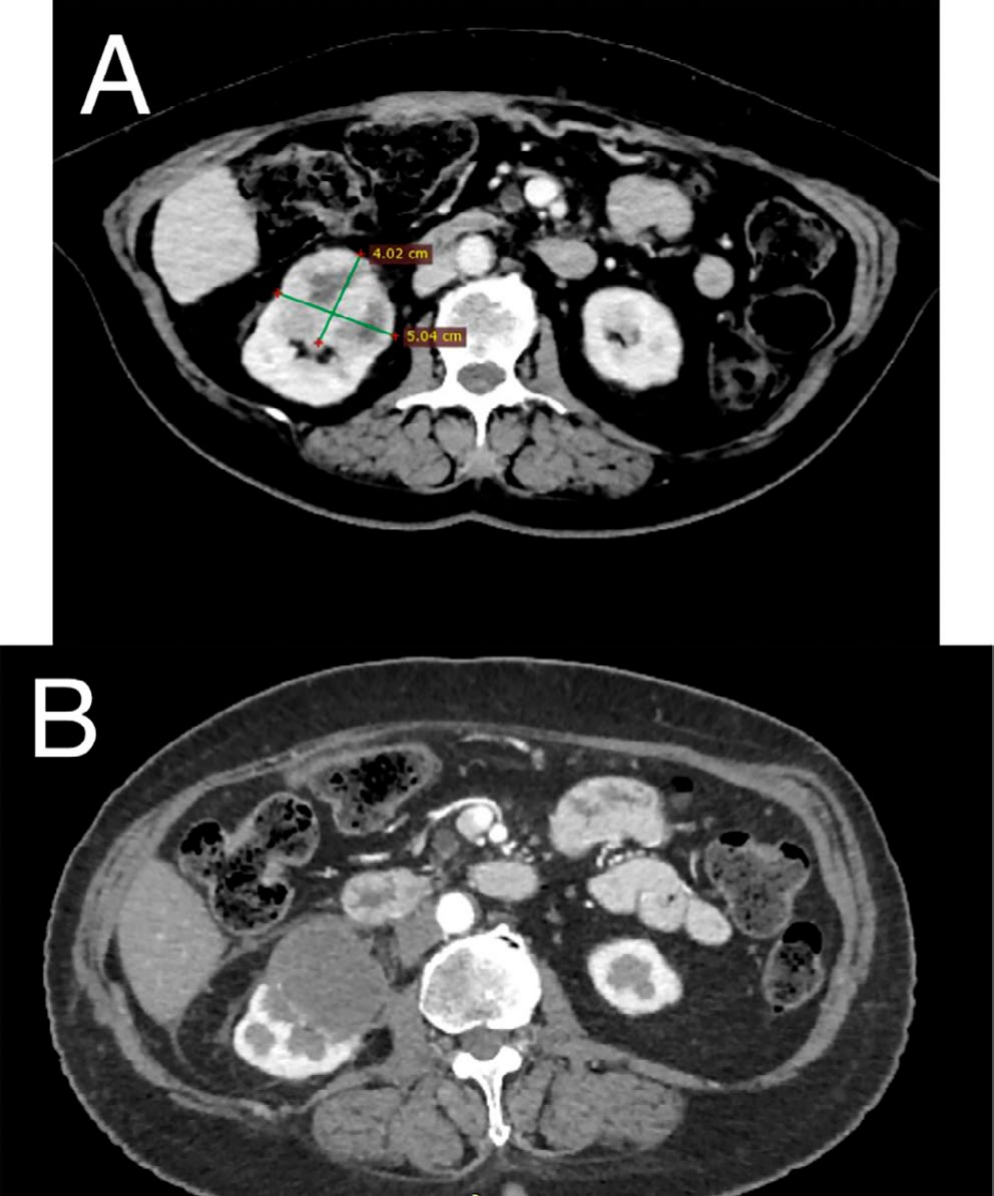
CT images before (A) and 6 months after (B) cryoablation procedure show complete tumor necrosis with no visible enhancement after contrast medium administration.
